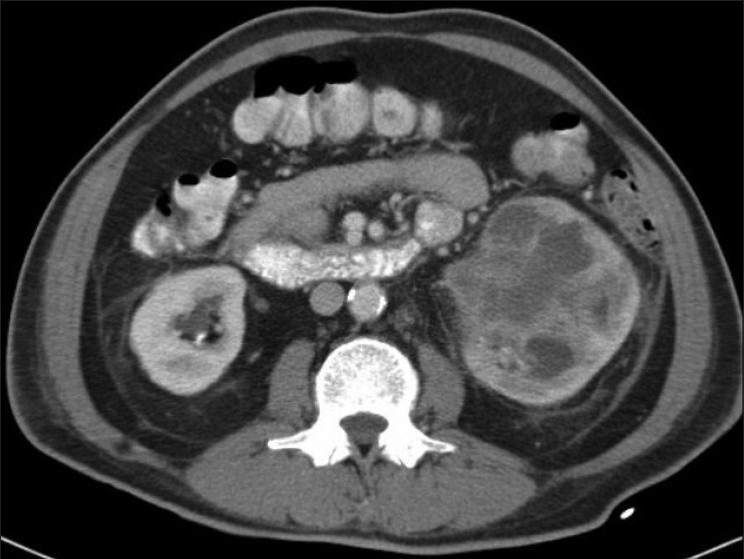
CT scan of the kidneys demonstrating large lower pole heterogenous mass with perirenal fat stranding
